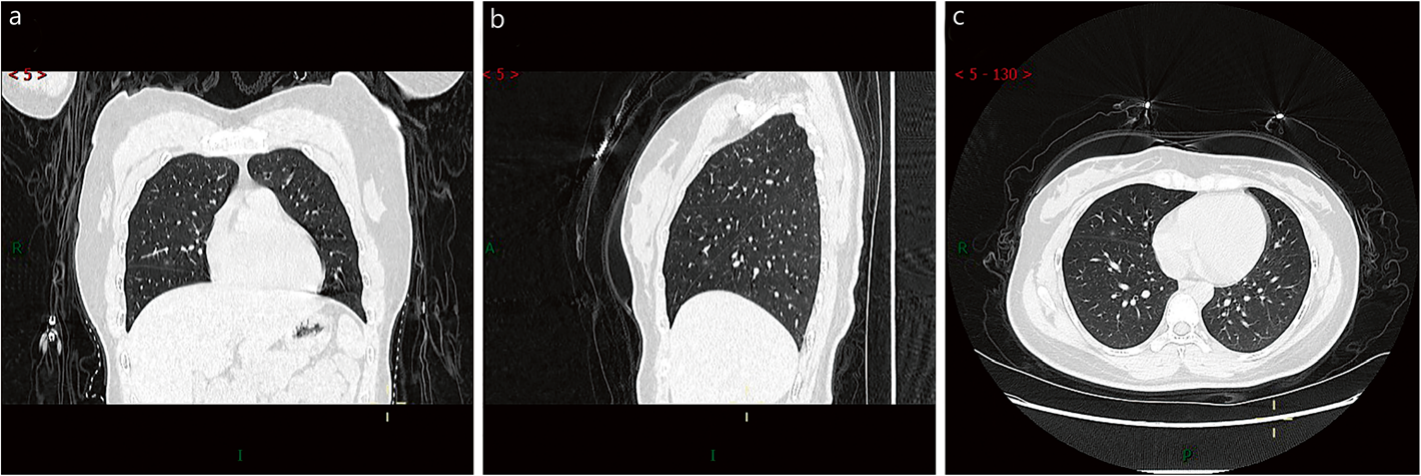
Chest CT of asymptomatic patient. A 27-year-old female with no clinical symptoms who had been in contact with COVID-19 patients was found to be positive for SARS-CoV-2 during screening. Patchy ground-glass opacities were seen in the lateral segment of the right middle lobe. Coronal (a), sagittal (b), and axial (c) sections in lung window. A: anterior; R: right
